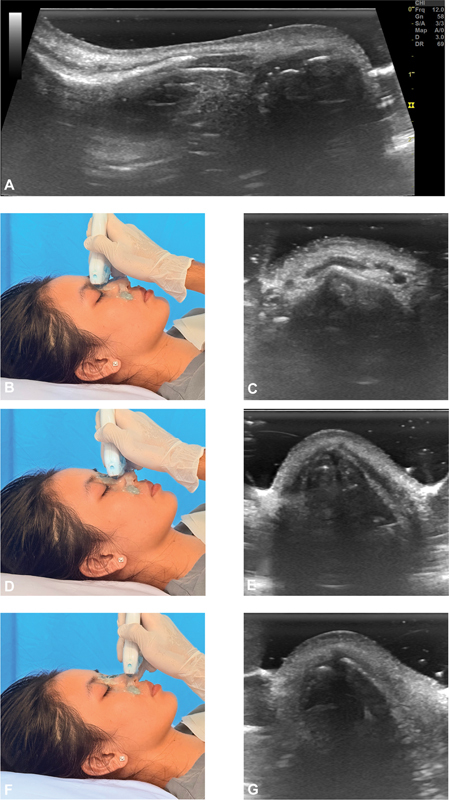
Ultrasound imaging of the nasal soft tissue envelope at three distinct nasal sites with corresponding probe positions. (A) Longitudinal scan along the nasal dorsum. (B, C) Axial scan and probe placement at the nasion. (D, E) Axial scan and probe placement at the rhinion. (F, G) Axial scan and probe placement at the nasal tip.
